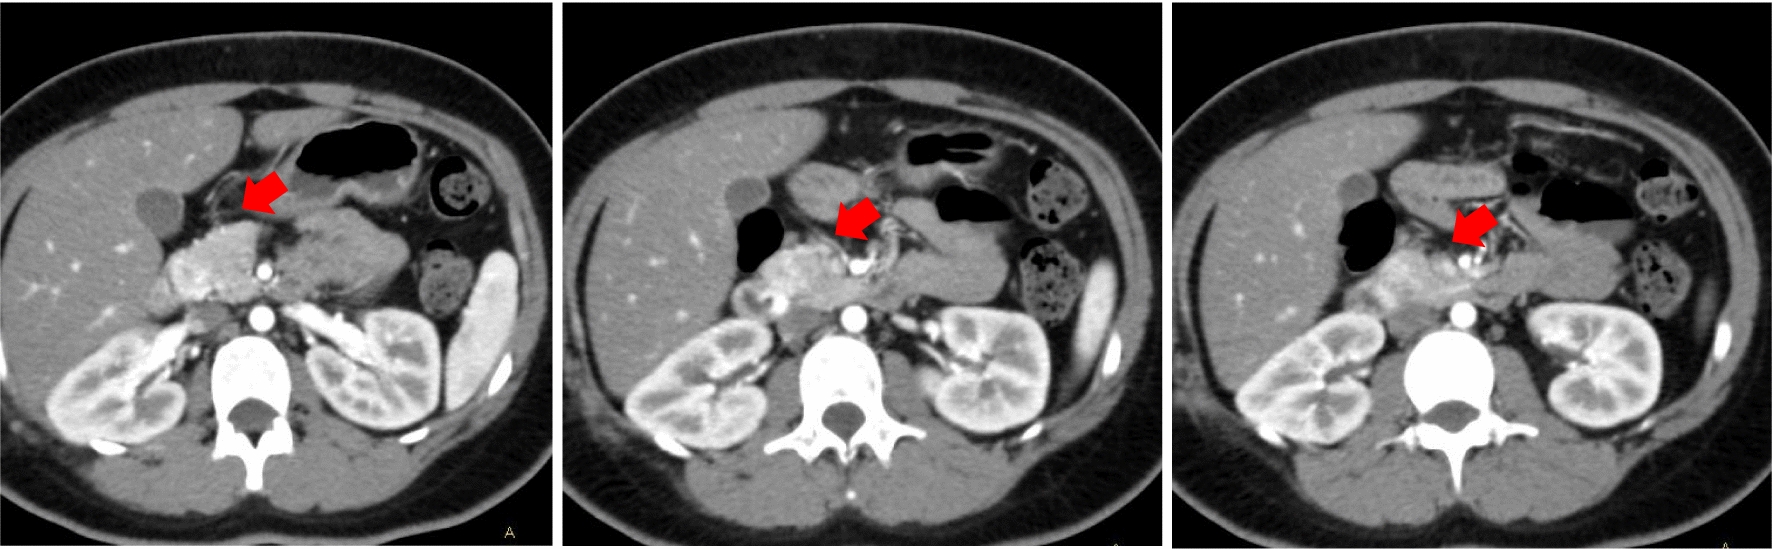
Transverse section CT scans indicated occupied lesion located in head of pancreas, which were labelled as red arrow. CT scans indicated that the tumor boundary was unclear and closely related to the surrounding organs. The enhancement was obvious. The pathological diagnosis was neuroendocrine tumor (NET) G2
